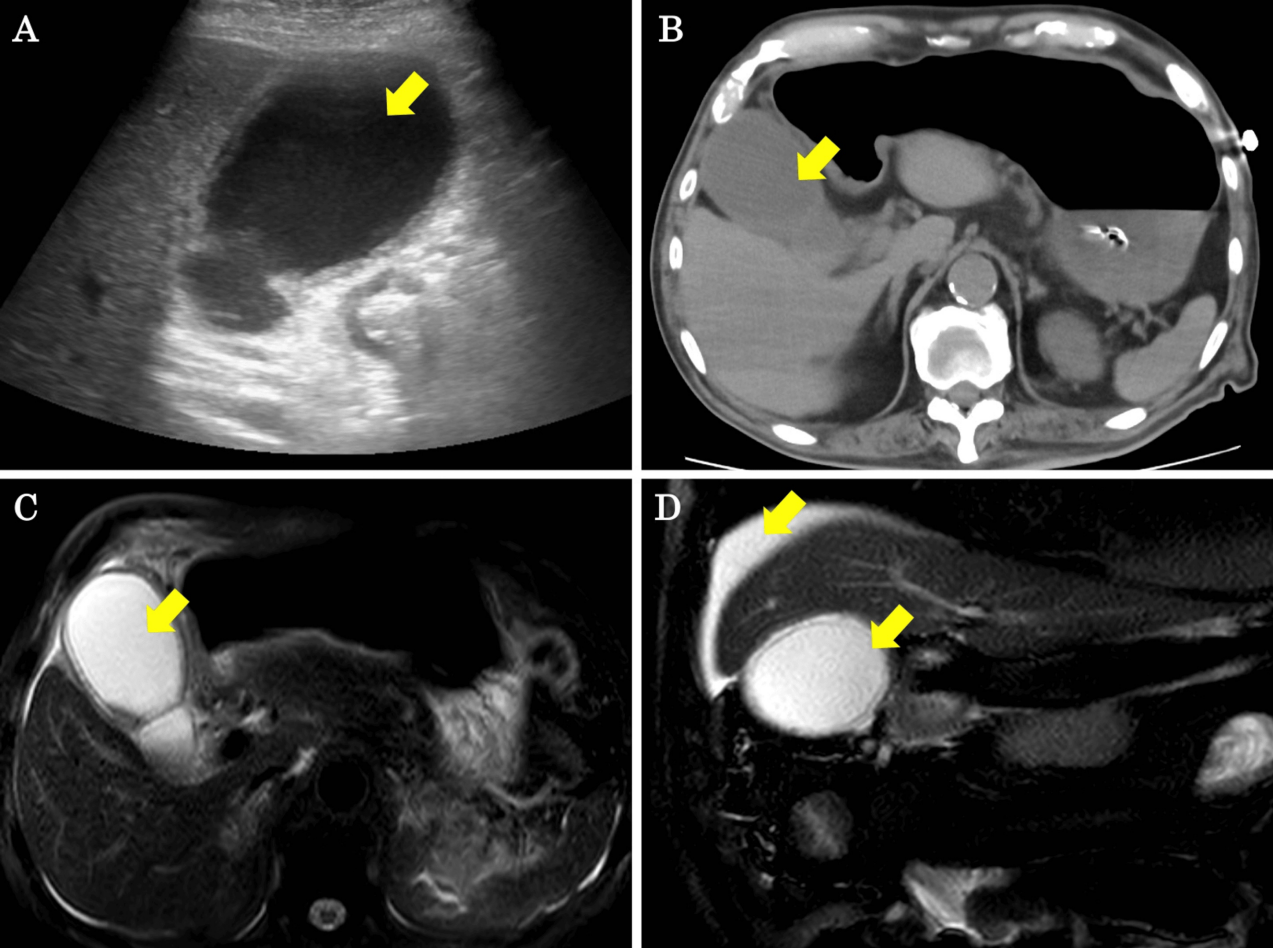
Abdominal ultrasound, CT, and MRCP findings on POD 6 (A, B) Abdominal ultrasound and CT show gallbladder enlargement, thickening of the gallbladder wall, and gallbladder debris (yellow arrows). (C, D) MRCP shows fluid accumulation around the gallbladder and on the surface of the liver, as well as edema of the gallbladder wall (yellow arrows). CT, computed tomography; POD, postoperative day; MRCP, magnetic resonance cholangiopancreatography
